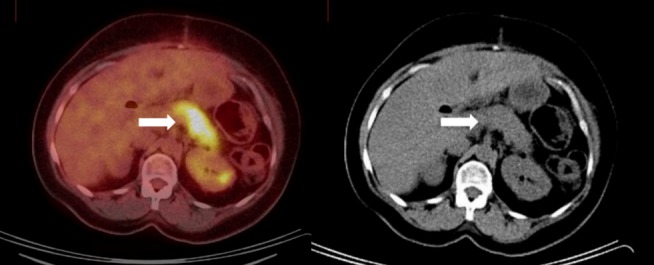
Postoperative initial PET/CT scan There is an FDG-avid lesion in the pancreatic tail on the PET axial image *(white arrow, left image) *with a corresponding hypodense correlate on the non-contrast enhanced CT axial image acquired as part of the same study *(white arrow, right image).*
